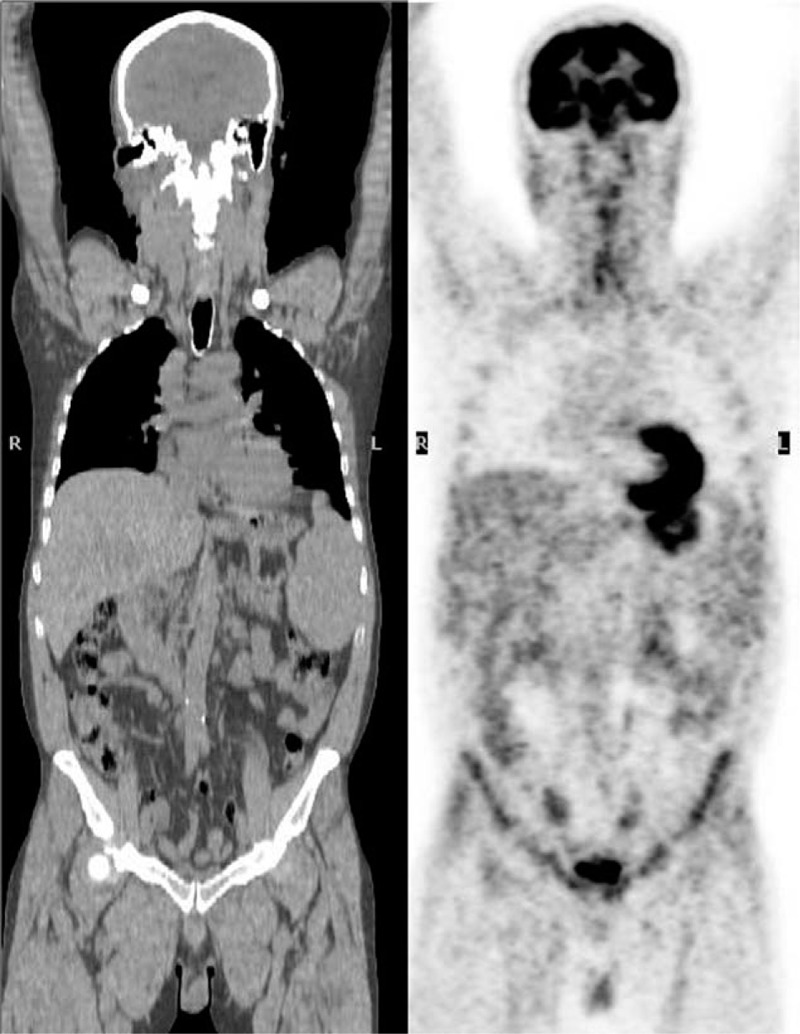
Reevaluation positron emission tomography/computed tomography scan revealed no fluorodeoxyglucose pathological accumulation, confirming clear disease regression.
